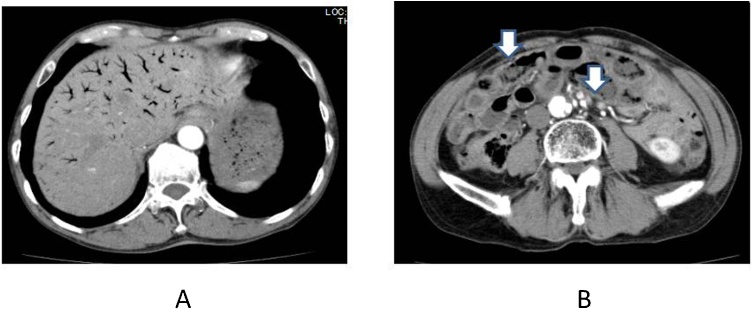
Contrast-enhanced CT on admission revealed hepatic portal vein gas in whole liver (1A) and intestinal pneumatosis at ileum (1B). White arrows indicate pneumatosis of intestine.
